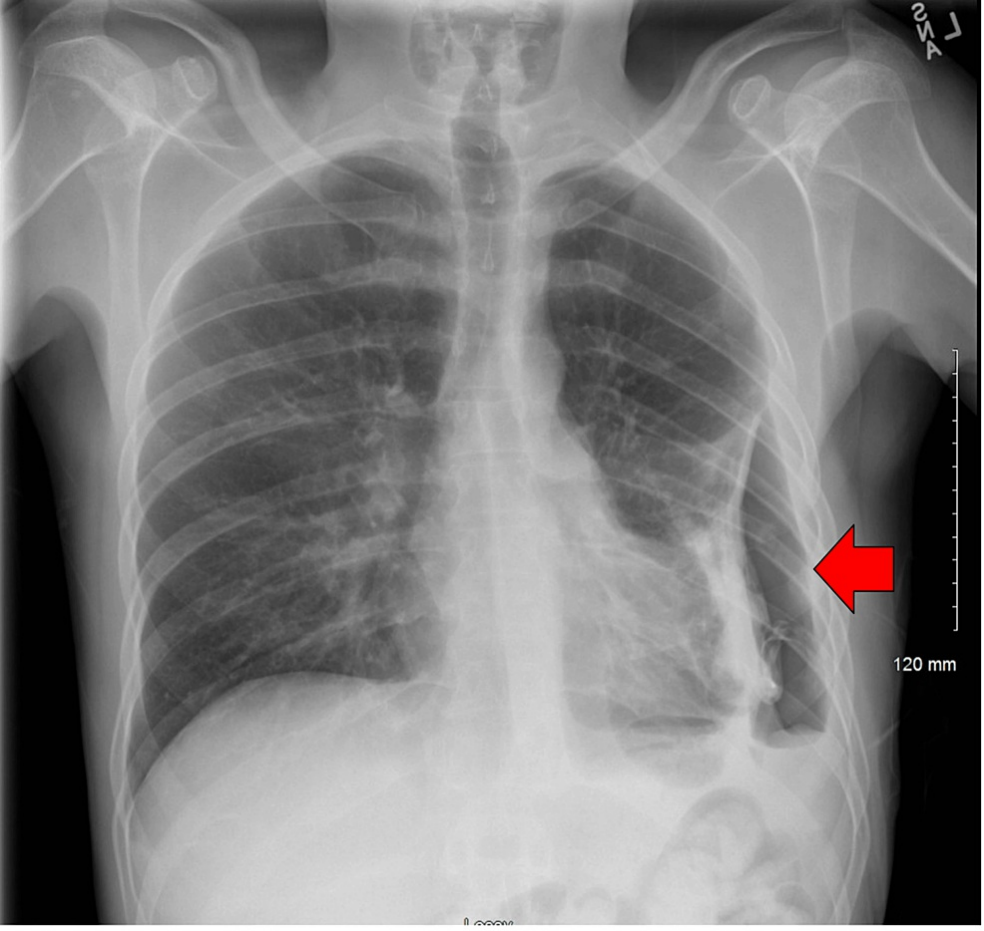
Chest X-ray three weeks post-discharge Chest X-ray showing stable left hydropneumothorax (red arrow) three weeks post-discharge.
